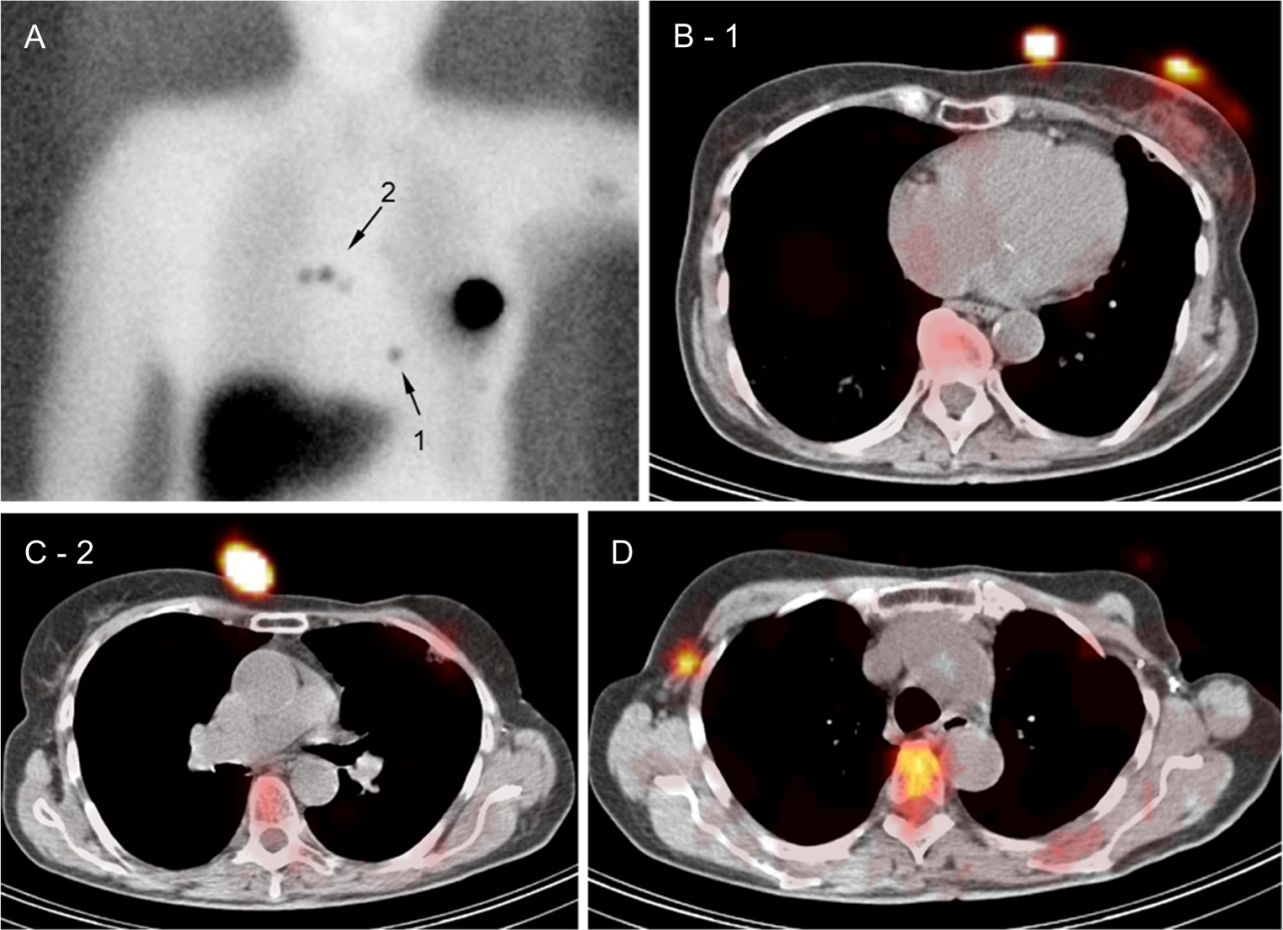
A 77-year-old woman with ductal carcinoma relapse in the left breast. Planar scintigraphy shows bilateral foci parasternally (a), arrows number 1 and 2. SPECT/CT (b) shows the first lower left focus as skin contamination. Also, the focus parasternally right appeared to be due to skin contamination (c). Note that a SN which was not visualized on planar scintigraphy is seen in level 1 of the right axilla (contralateral) on SPECT/CT (d). This axillary SN was negative for metastasis
